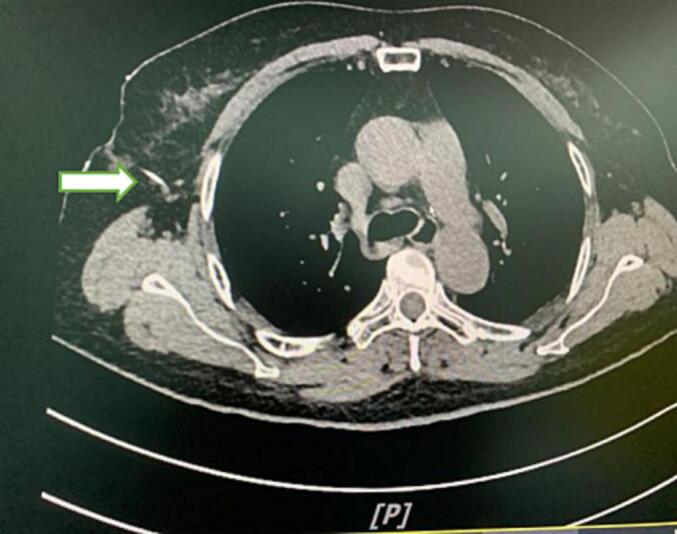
Chest computed tomography displaying the steel wire in the thoracic cavity (arrow).
